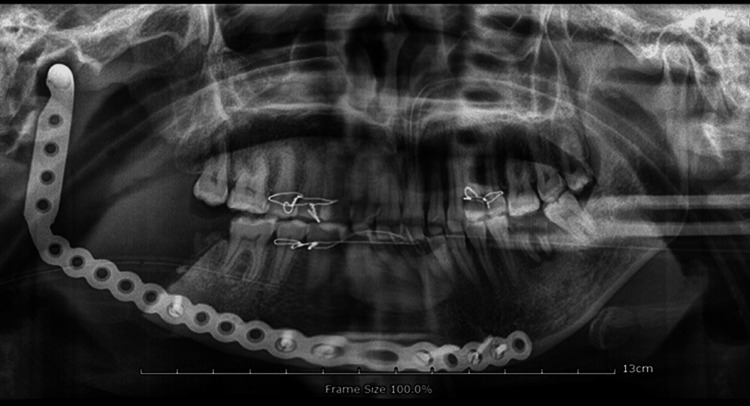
Post-operative OPG showing the fixed reconstruction plate OPG - Orthopantomogram
